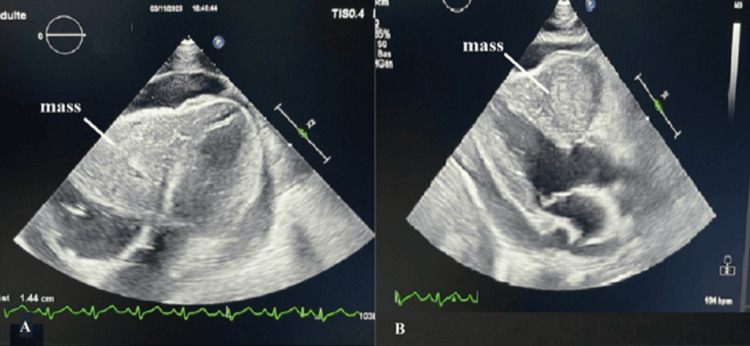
Echocardiographic appearance of the mass - (A) apical four chamber view and (B) parasternal long axis view.
